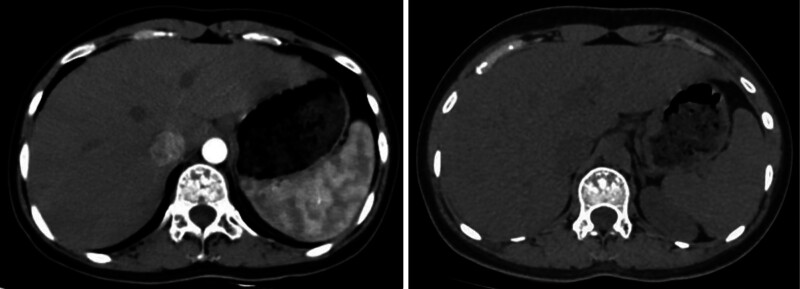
Abdominal enhanced computed tomography (CT) in July 11, 2020, and abdominal CT plain scan in July 21, 2023.
